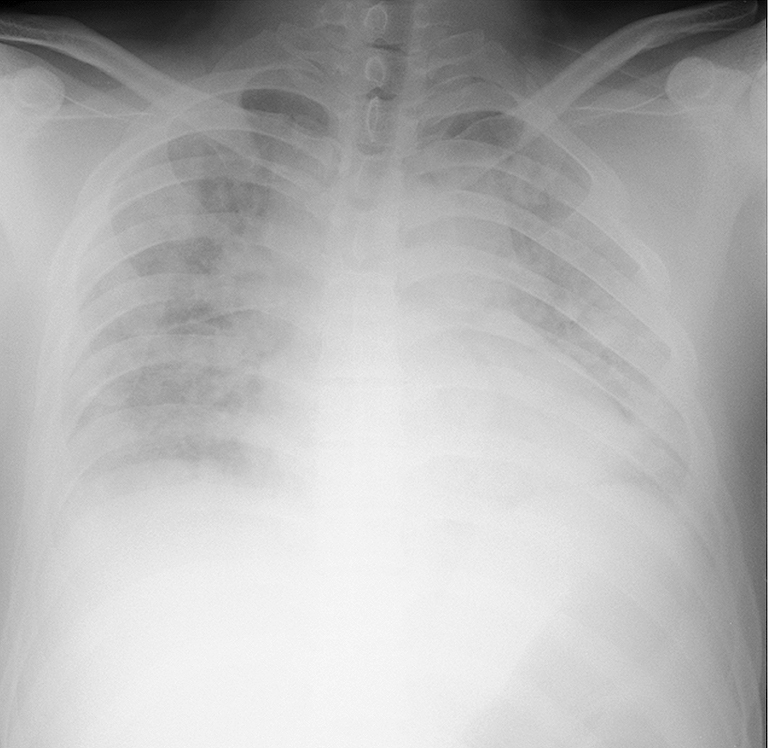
Chest radiography revealed bilateral alveolar shadows.
